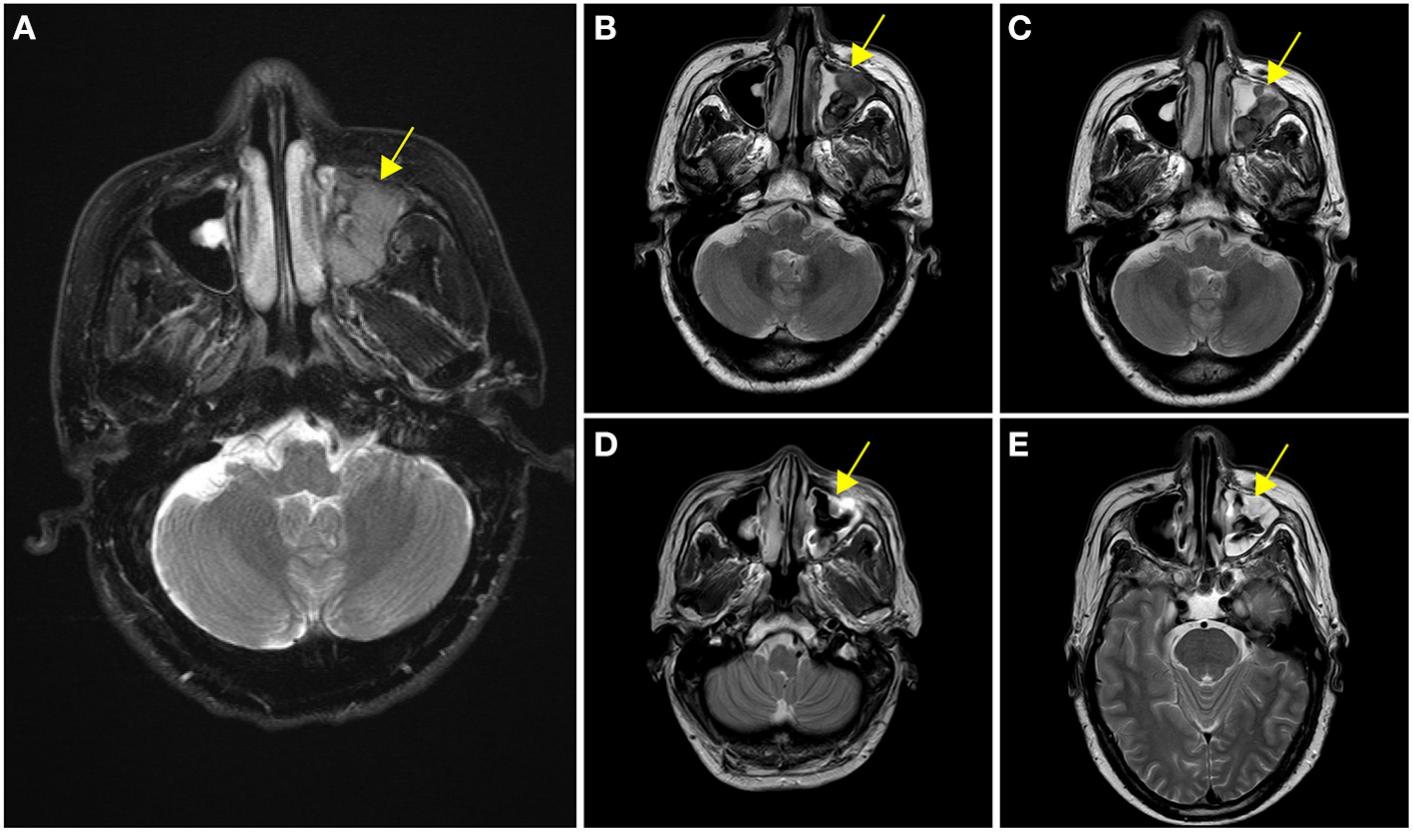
Enhancement of nasopharyngeal MRI scanning at different stages of treatment. (A) Initial enhancement MRI scanning revealed heterogeneous enhancement shadow filling in the left maxillary sinus at diagnosis. (B) After two cycles of V-CDOP chemotherapy, enhanced MRI scanning showed an excellent initial response with a substantial decrease in the size and intensity of nasopharyngeal lesions. (C) After four cycles of V-CDOP, MRI scanning revealed no significant change. (D) After radiotherapy and two cycles of RV-CDOP, subsequent enhancement MRI scanning revealed a reduction in the size of nasopharyngeal lesion. (E) Repeated enhancement MRI scanning after autologous hematopoietic stem cell transplantation (ASCT) showed increased abnormal signal shadows in the maxillary sinus, which was considered an inflammatory reaction. The nasopharyngeal lesions were indicated by yellow arrows.
